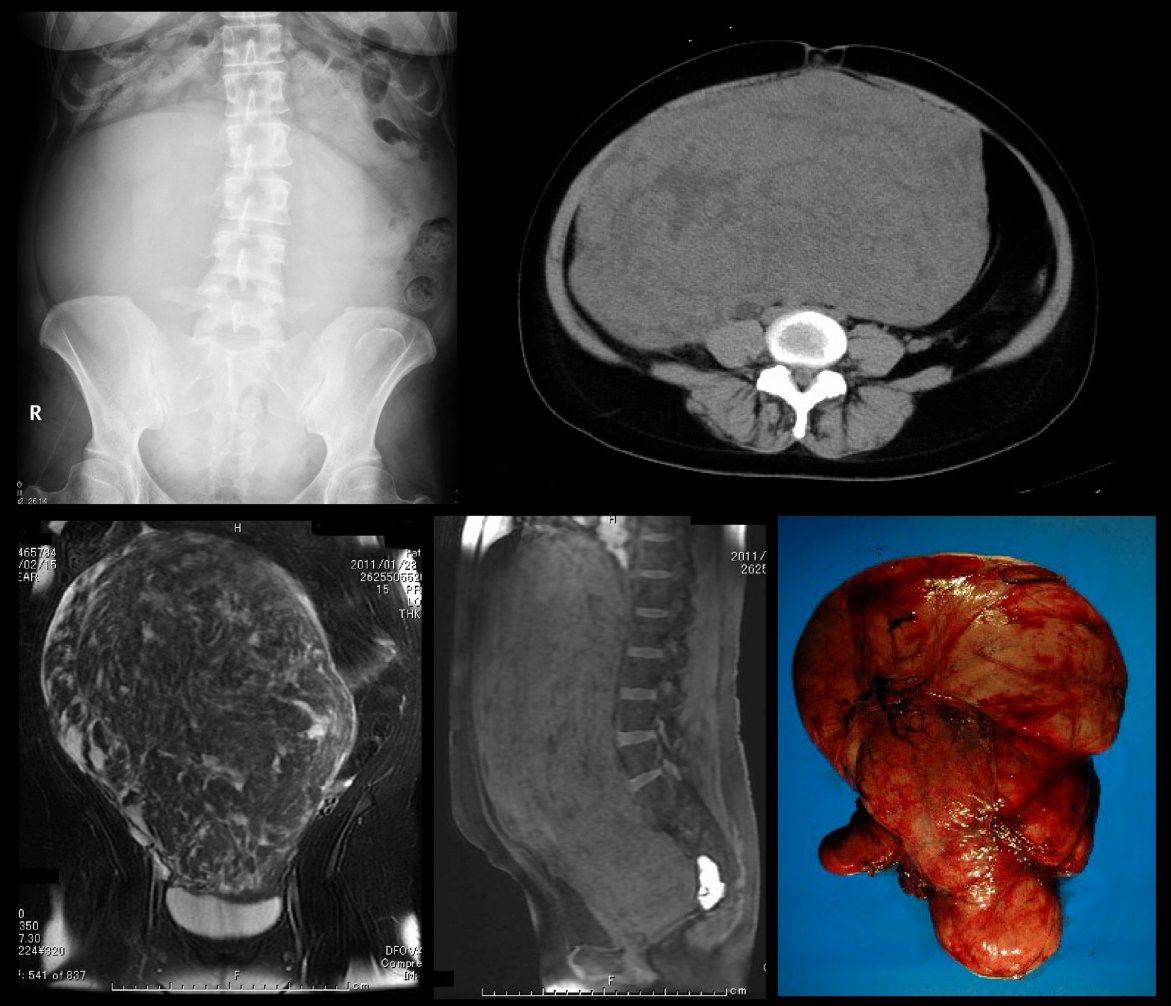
A giant uterine leiomyoma on an abdominal X‐ray film (upper left panel) and computed tomographic (upper right panel) and magnetic resonance images (lower left and middle panel). A macroscopic view is shown in the lower right panel.
